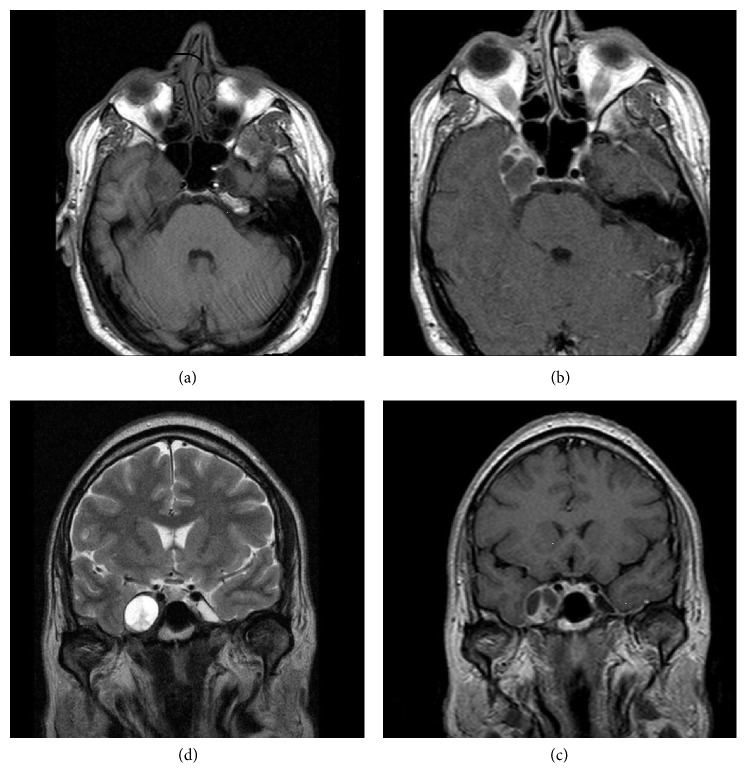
One can see clockwise the MR images of the tumour on T1-weighted, on T1 with contrast, and on T2-weighted images.
